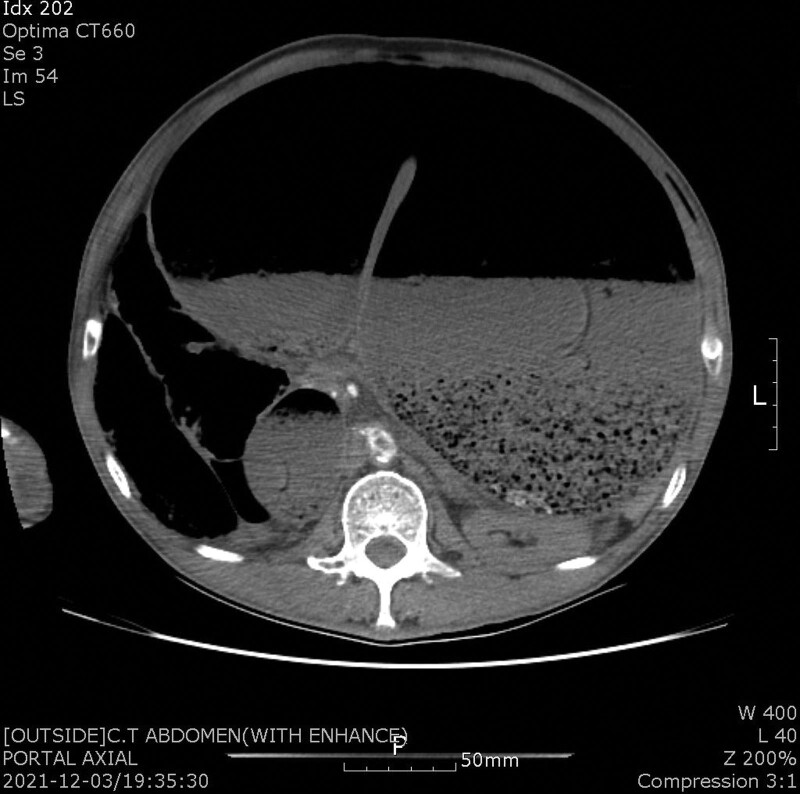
Axial computed tomography image of the patient, showing critical abdominal distension with severe gastric dilatation were noted, in addition to compression of the abdominal aorta and suspicious distal thrombosis.
